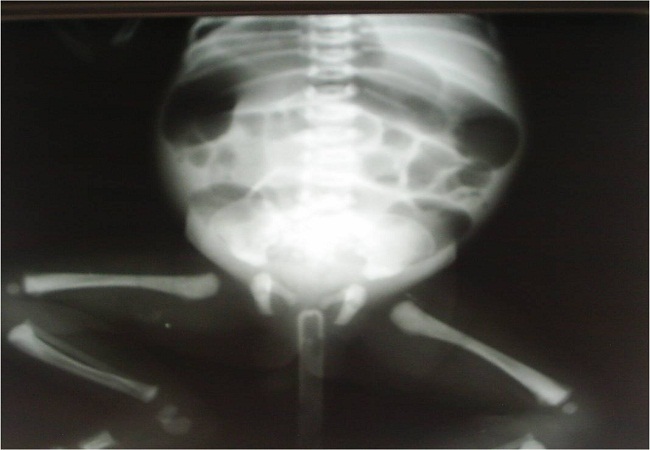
Radiographic X-Ray revealed dilated bowel loops but no air-fluid levels or pelvic gas
